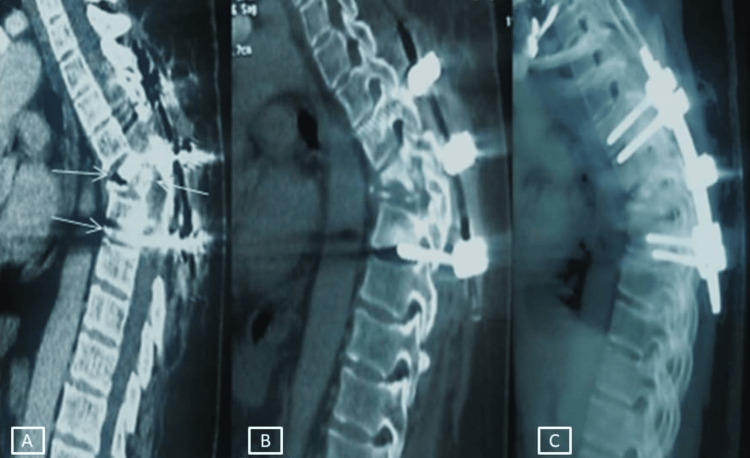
Computed tomography of the dorsal (thoracic) spine showing sequelae of spondylodiscitis: D5-D6 dorsal kyphosis Post-surgical changes following decompressive spinal laminectomy, demonstrating worsening of thoracic kyphosis with osteosynthesis.
